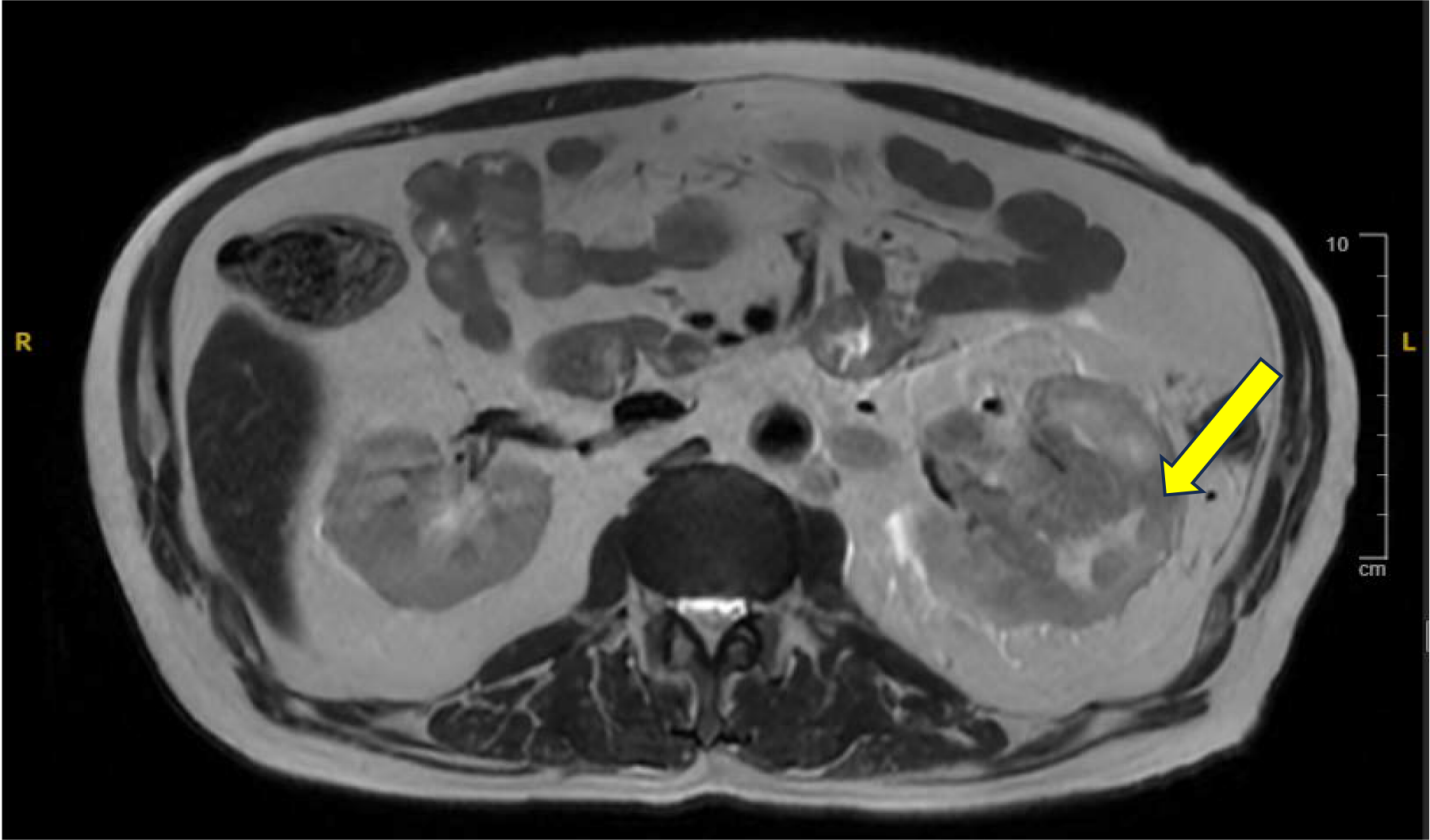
Axial contrast-enhanced MRI showing a heterogeneous enhancing mass in the left kidney (arrow), subsequently confirmed as renal cell carcinoma with sarcomatoid differentiation on biopsy.
